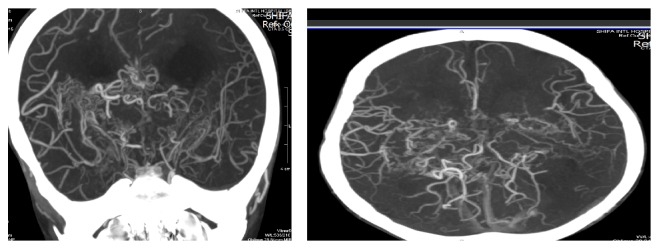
Postoperative CTA head showing good collateral supply to both cerebral hemispheres.
